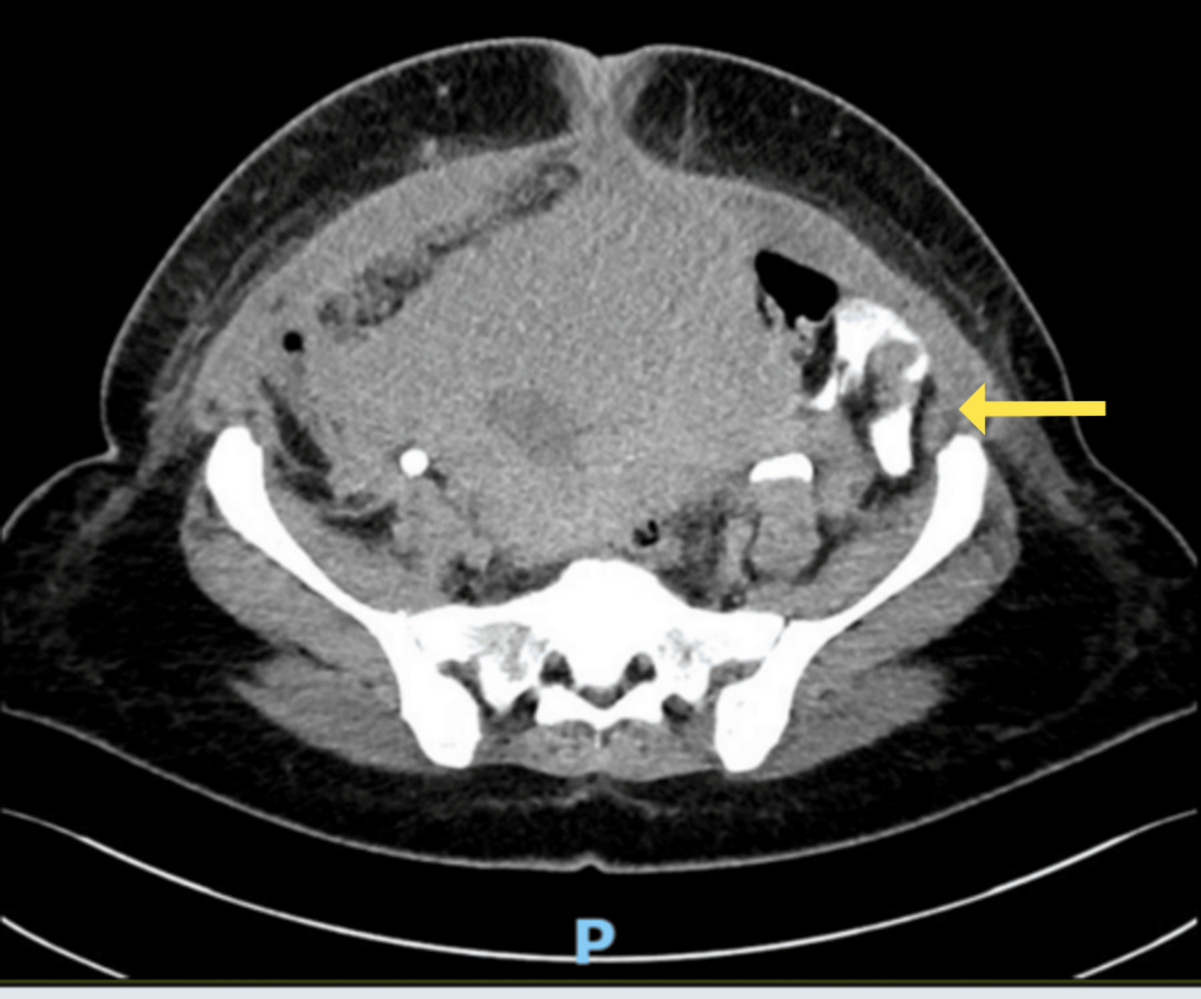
Axial view of the initial CT cystogram demonstrating extravasation of contrast that is spreading within the peritoneal cavity between the bowel loops (yellow arrow).
